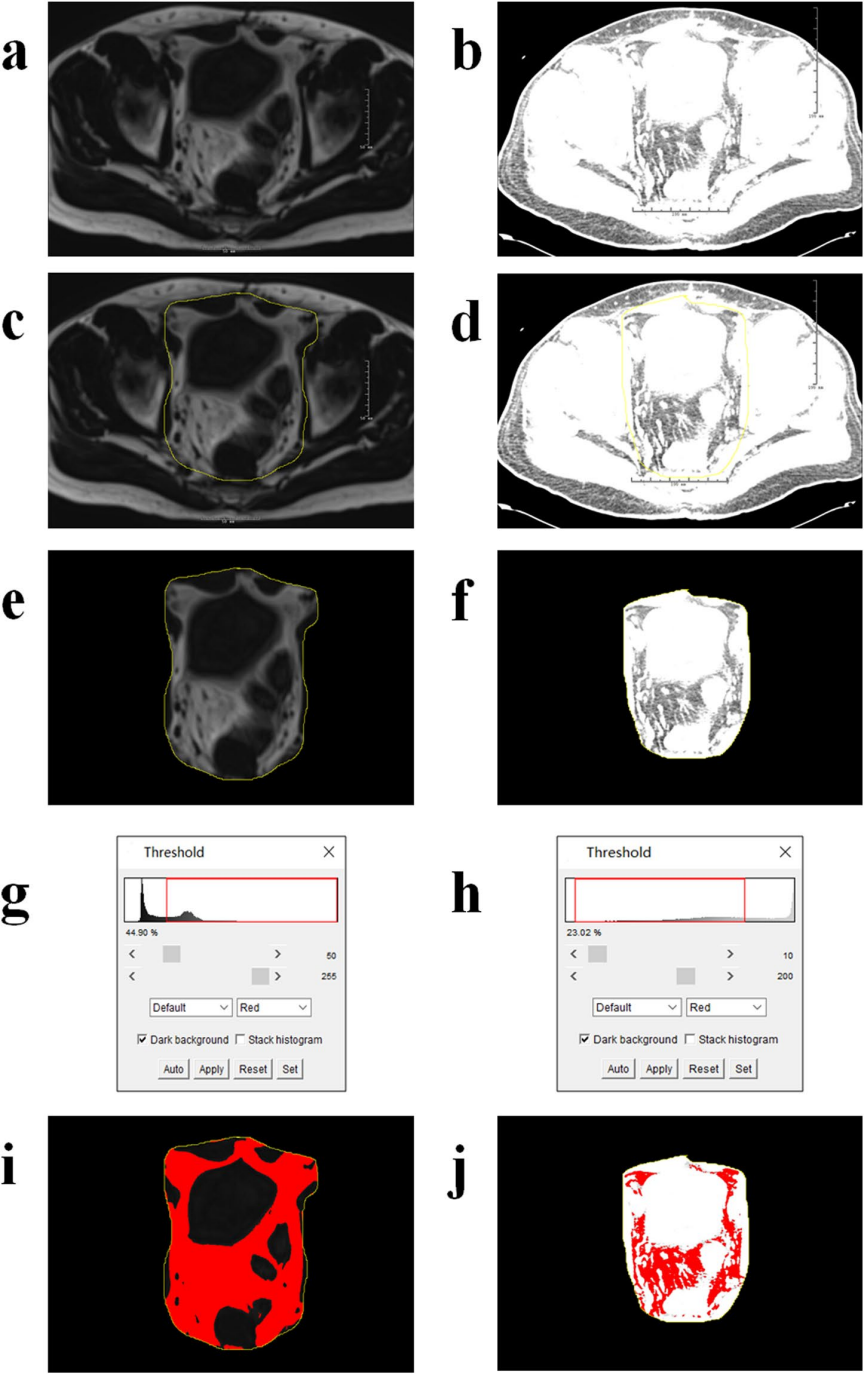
Process of measuring AreaM using both pelvic MRI-DXION-F images (a,c,e,g,i) and pelvic CT images (b,d,f,h,j) in the same patients. Circle peripouch fat (c,d), clear outside irrelevant regions (e,f), set thresholds (g,h), and finally calculate peripouch fat area (i,j).
